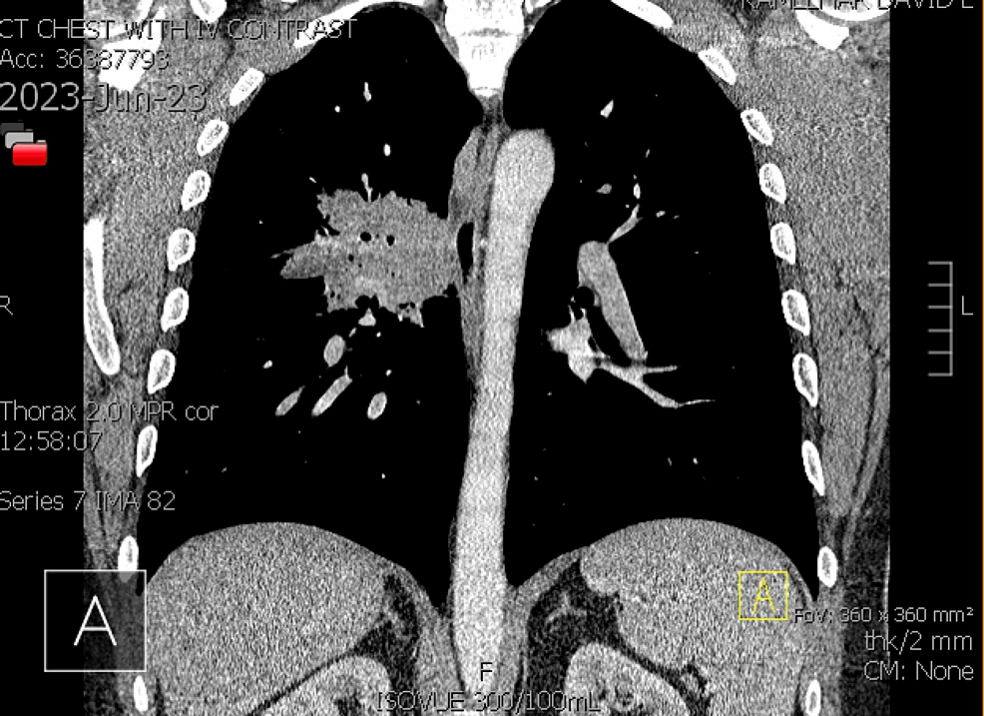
CT chest with IV contrast showing a lobulated right upper lobe mass (coronal view)
